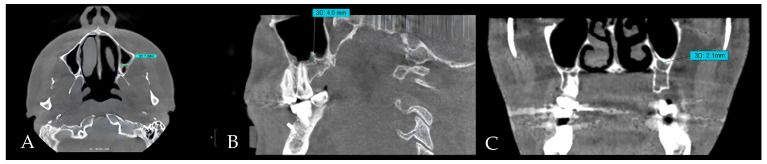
CBCT images of the maxillary sinus septa. (A) Mediolateral diameter of the maxillary sinus septa depicted on the axial plane. (B) The height of the observed septa measured on the sagittal plane. (C) The thickness of the observed septa measured at its thickest part.
